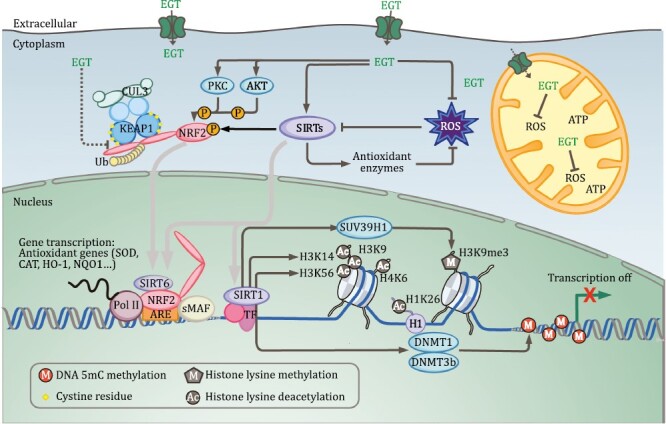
Interaction of Ergothioneine with KEAP1–NRF2 and Sirtuin pathways. Ergothioneine regulates KEAP1–NRF2 complex, as well as SIRT1 and SIRT6 expression levels, to upregulate antioxidant genes, such as superoxide dismutase (SOD), catalase (CAT), heme oxygenase-1 (HO-1) and NAD(P)H:quinone oxidoreductase (NQO1). SIRT1 deacetylates NRF2, histone H1, H3, and H4, and modulates the activity of certain epigenetic enzymes, including methyltransferase SUV39H1 for histone H3K9me3 trimethylation, DNMT1 and DNMT3b for DNA 5mC methylation.
